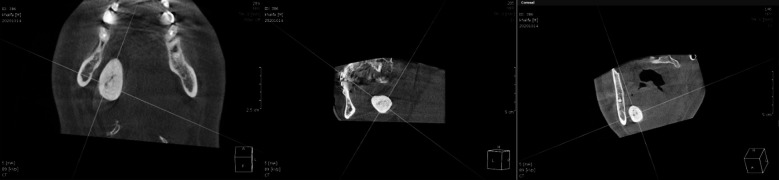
Axial, Sagittal and Coronal CBCT view reveals the concentric circular layers of calcification around the central nidus.
